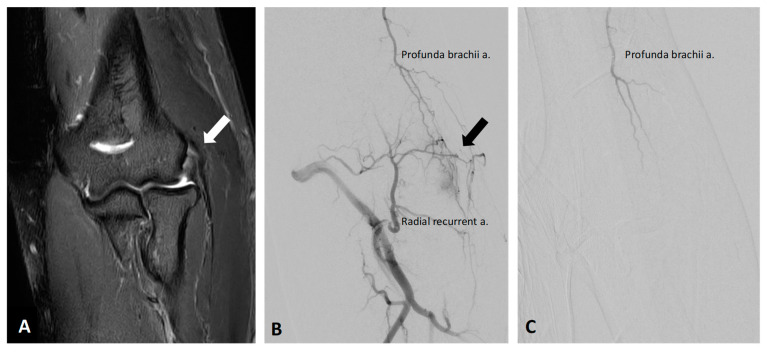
Representative pre- and postprocedural images of the right elbow lateral epicondylitis in a 58-year-old male. (A) Preprocedural coronal MRI (fat-saturated T2-weighted image) revealed abnormal thickening with elevated signal intensity within the common extensor origin from the lateral epicondyle (white arrows). (B) Preprocedural angiography of the right radial recurrent artery showed angiogenesis with reflux to profunda brachii artery (black arrows) over the corresponding enhanced region of MRI. (C) Postembolization angiography via profunda brachii artery and other arteries indicated the elimination of angiogenesis after TAE. The patient’s lateral elbow pain and function both were improved after TAE.
